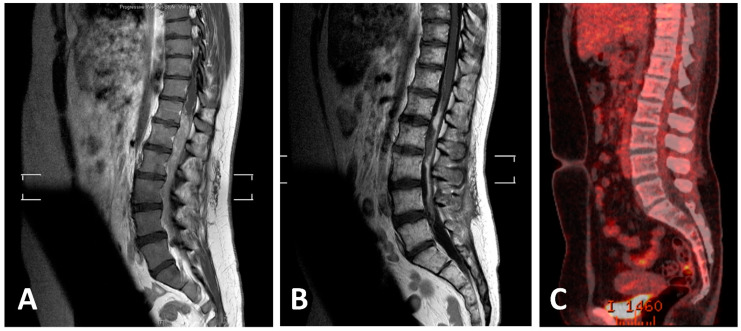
MRI and PET-CT scans of lumbar EwS before and after definitive RCth (radiochemotherapy) and ASCT (autologous stem cell transplantation). (A) Initial T1-weighted sagittal MRI of the thoracic and lumbar spine with intramedullary tumor; (B) T1-weighted sagittal MRI with residual post-therapeutic signal alterations; (C) post-therapeutic PET-CT scan with no residual increased FDG uptake.
